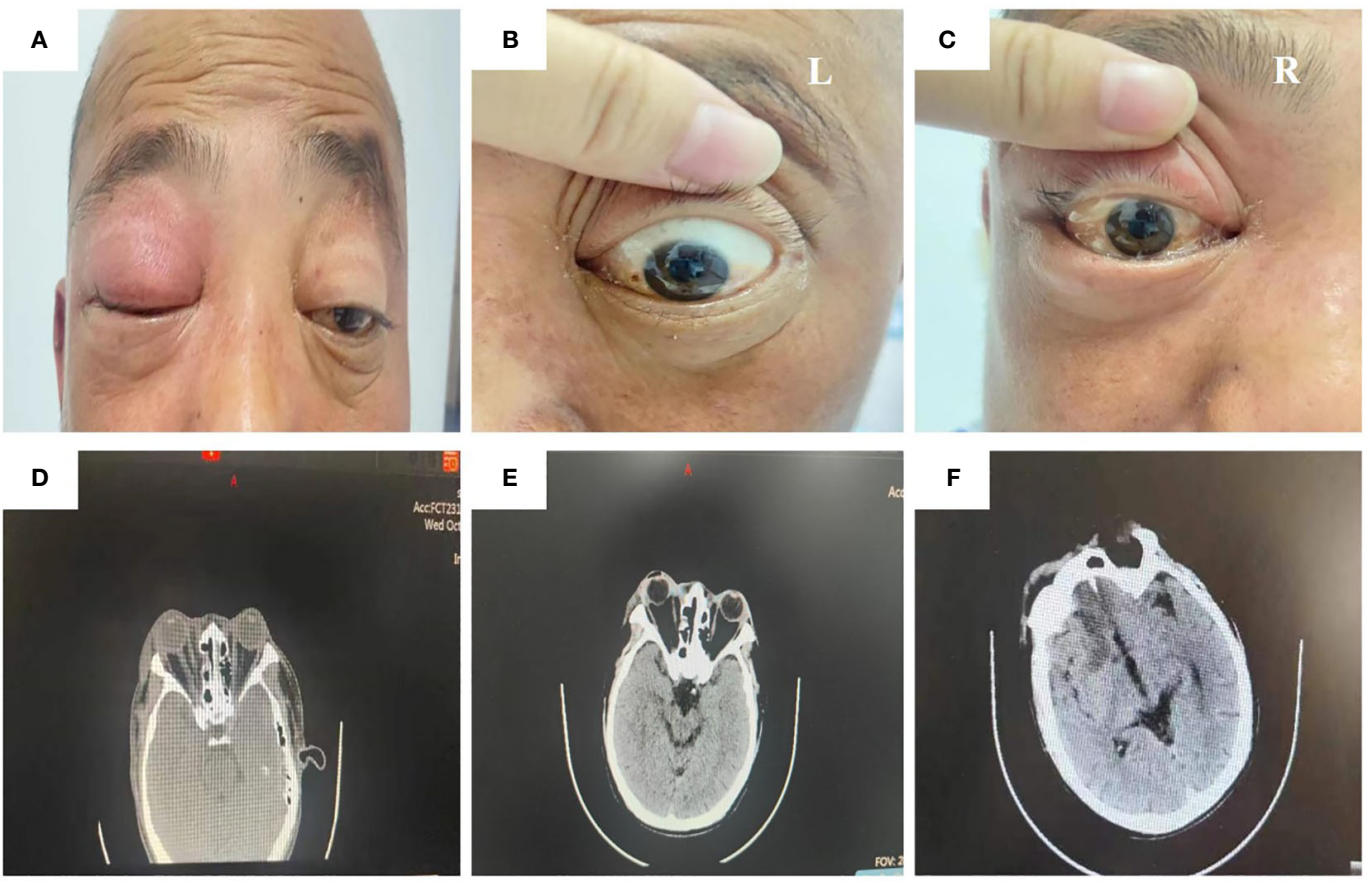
Physical examination and CT image of the patient during administration. The ocular signs of physical examination on day 1 after admission (A–C). The orbital CT image on day 1 after admission showed bilateral maxillary and ethmoid sinusitis (D). The head CT image on day 3 after admission showed swelling of soft tissue around the right orbit, slightly aggravated compared to the first day after admission (E). The head CT image on day 4 after admission showed a low-density shadow in the bilateral frontal lobe and right anterior horn of the lateral ventricle (F).
